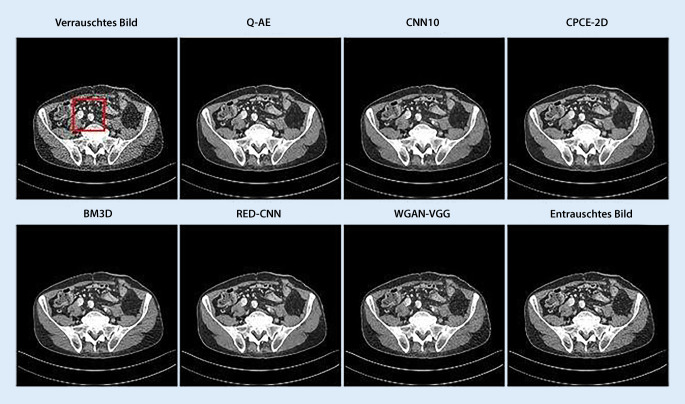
Ein umfassender Überblick über die Methoden findet sich in [2]. Neben den vielversprechenden Ergebnissen der Methoden zur Entfernung des Rauschens aus Niedrigdosis-CT-Bildern werden inzwischen Modelle eingeführt, die effizienter als früher verwendet werden.
